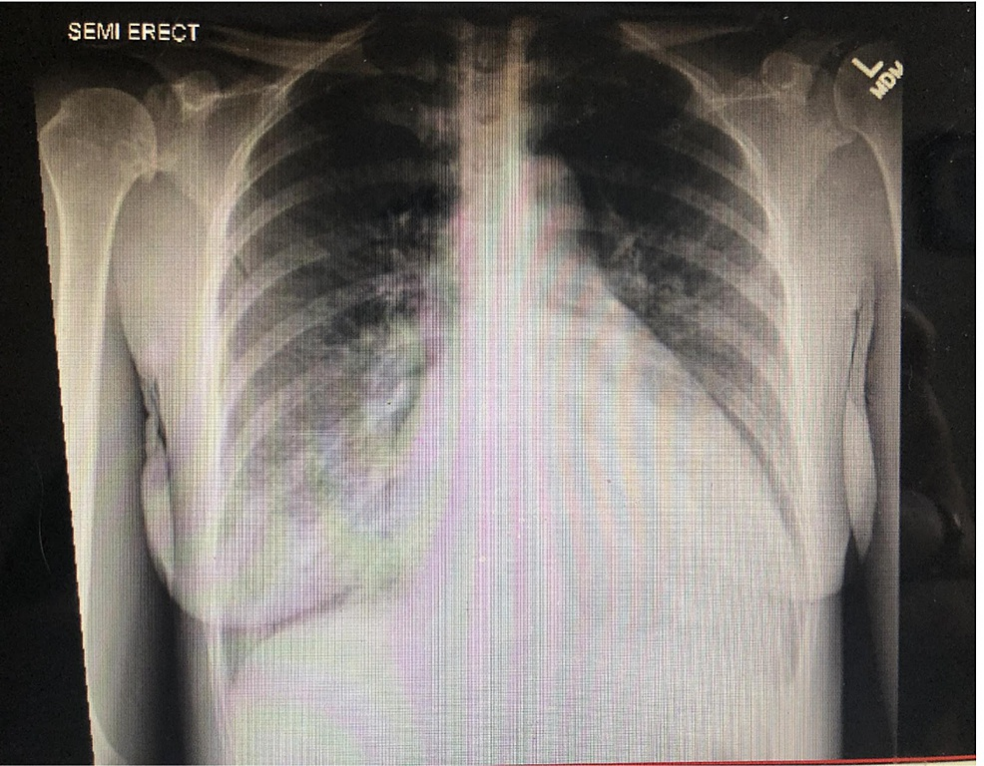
Standard posteroanterior chest X-ray CXR of a 47-year-old female who presented with one week of shortness of breath, cough productive of white blood tinged sputum, nausea, diarrhea, diaphoresis, orthopnea, palpitations, chest pain, and dyspnea on exertion. The CXR showed cardiomegaly and pulmonary edema.
